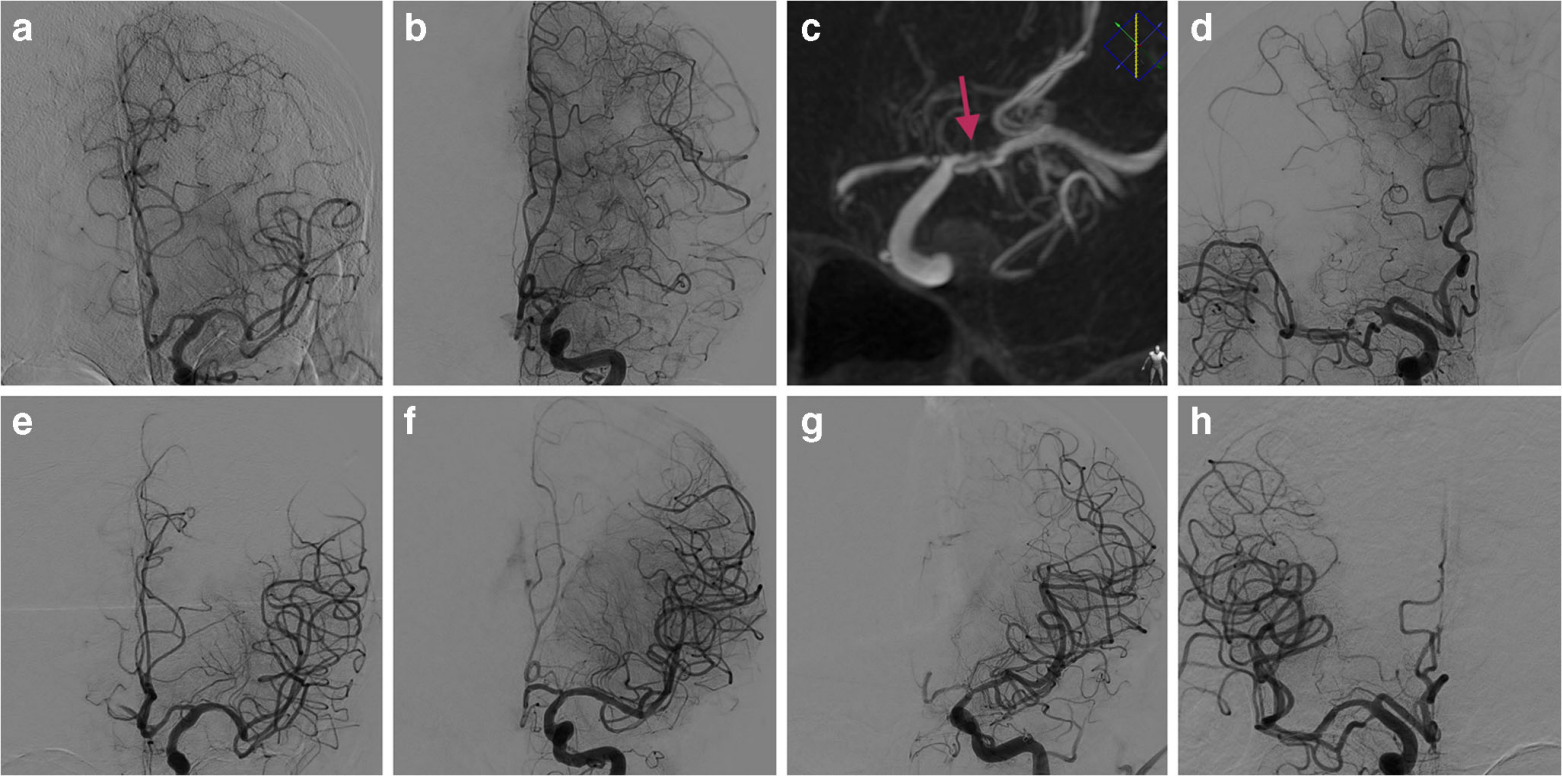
Cases 1/2/3/4. a, b, and d Angiographic aspect of middle cerebral artery stenosis before rescue stenting. c Cone-beam CT angiography showing a dissected plaque (red arrow). e–h Angiographic control after stenting
